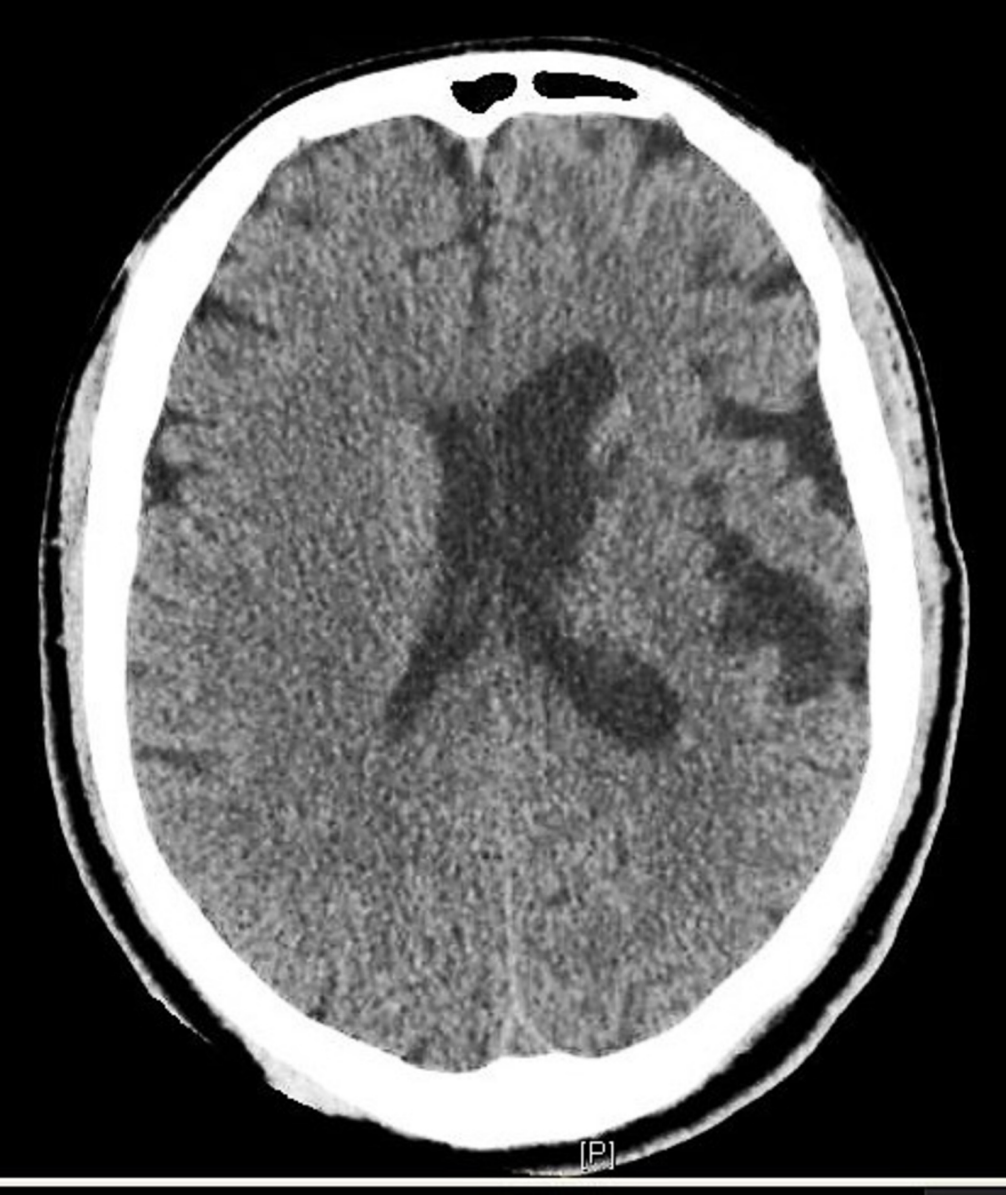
Computed tomography (CT) scan head without contrast showing ex vacuo dilatation of left lateral ventricle.
